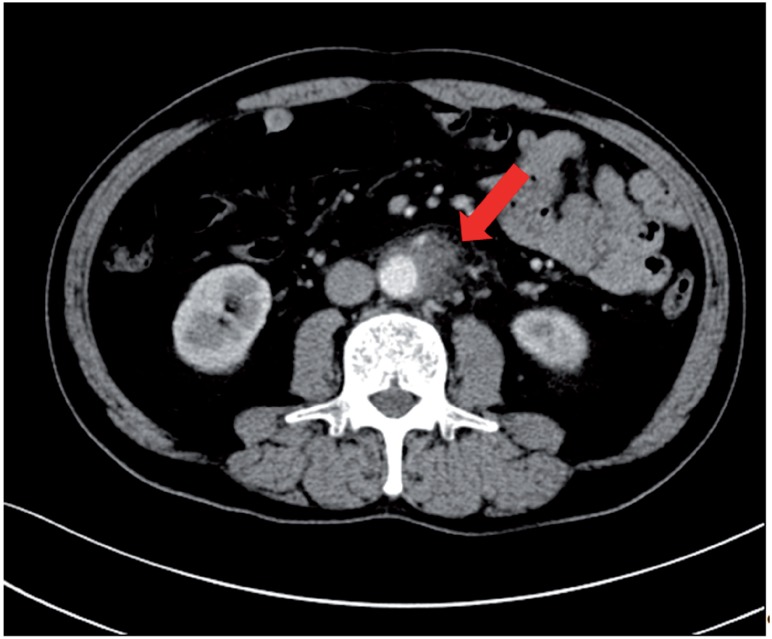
Enhanced computed tomography revealed a low density area surrounding the aorta at the level of the inferior mesenteric artery and the right total iliac artery.
